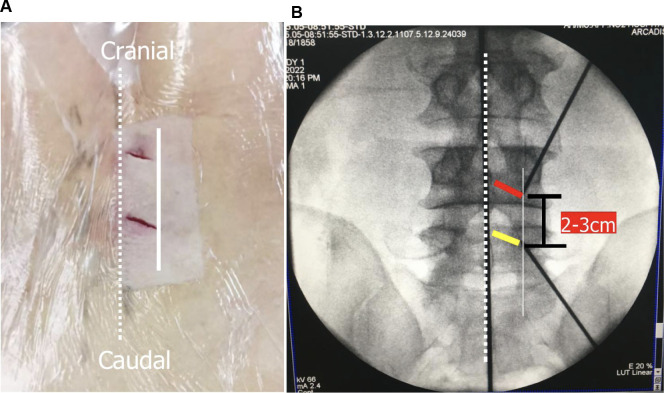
Initial placement of two portals and related anatomy. (A). Skin entry points for two portals; and (B) position of two portals on an anteroposterior view of x-ray (yellow line: the site for placement of the cranial portal; red line: the site for placement of the caudal portal; dotted line: midline; white line: medial pedicular line).
